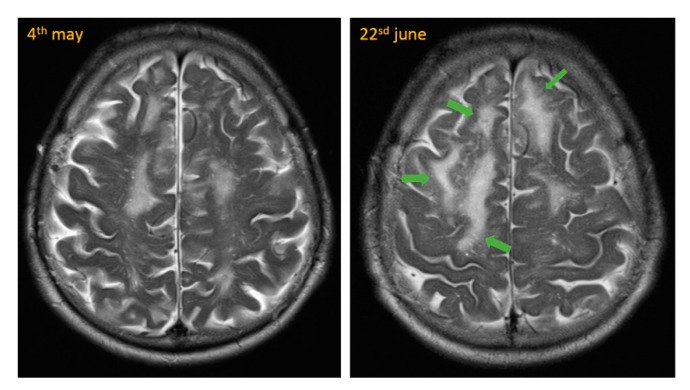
MRI with progressive and new white matter oedema (green arrows) in T2-weighted images.
